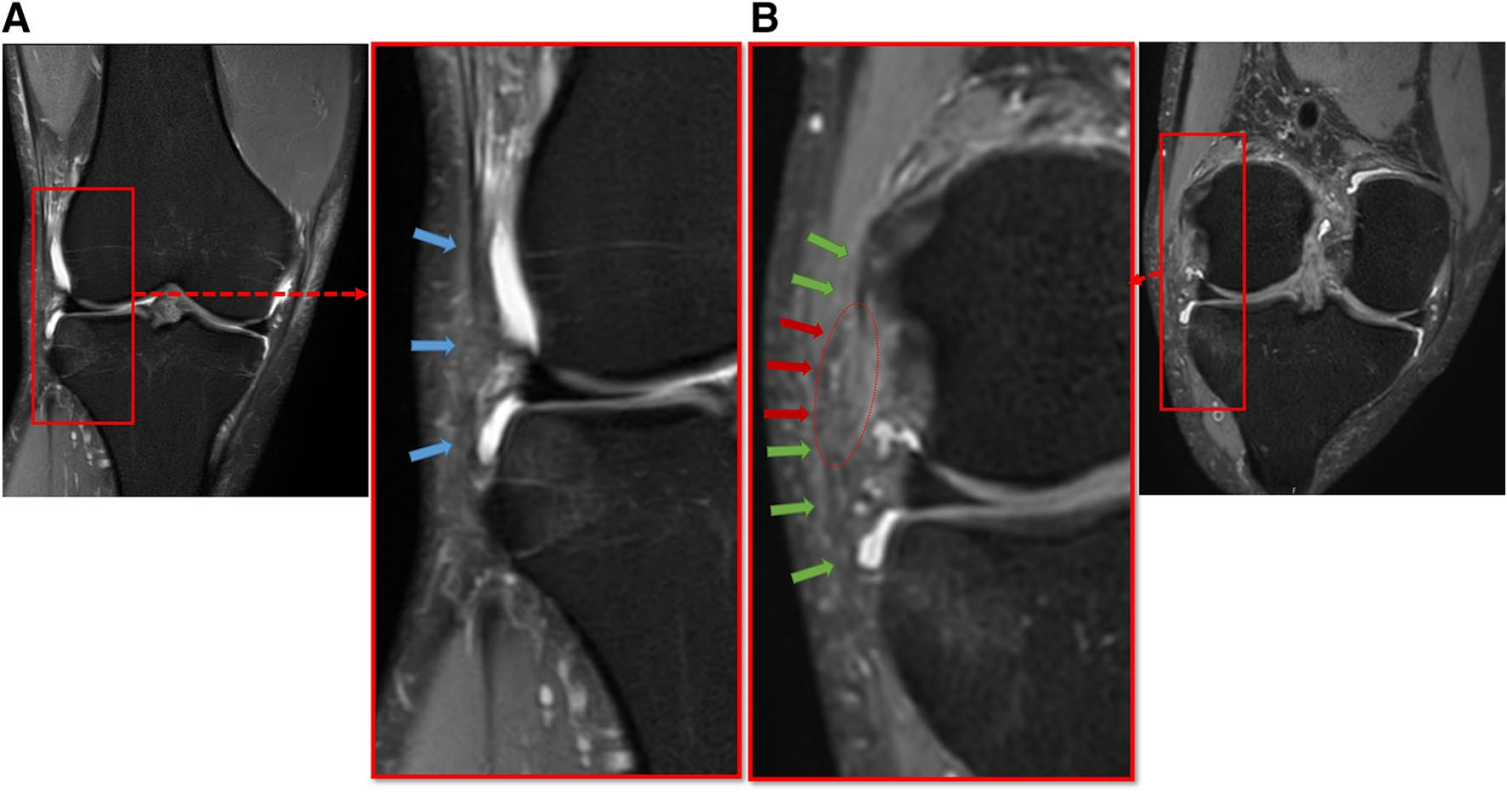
An example demonstrating the advantages of MPR MRI images. The ALL cannot be distinctly visualized as a continuous structure from its origin on the lateral femora to its attachment on the lateral tibia based on SCR images (A). Judgement based on these SCR images is difficult and raters judged the ALL as “strained”. However, it is clearly visible based on the MPR images (B) and raters judged it as torn. On the right, the red arrows indicated the torn part of ALL, green arrows indicate the remnants of the ALL. On the left, the blue arrows indicate the suspected ALL on the SRC image
